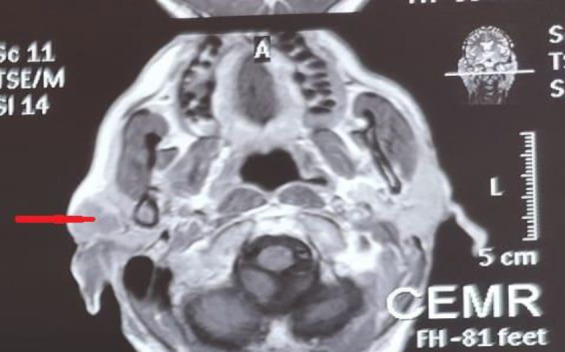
Contrast MRI neck: Axial post contrast T1 Weighted sequence of the neck showing Right side of parotid mass (Red arrow). The lesion appears mildly hypointense on T1-weighted images with mild peripheral enhancement.
